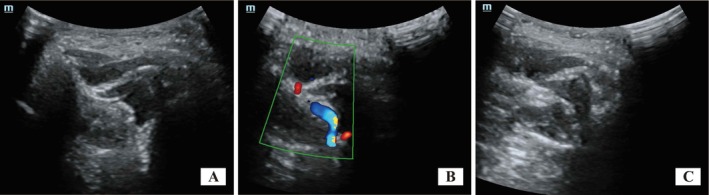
Ultrasound‐guided SPG block. (A) Anatomical view of the sphenopalatine fossa (white arrow). (B) Position of the maxillary artery (red arrow). (C) Ultrasound image of needle insertion (yellow arrow).
